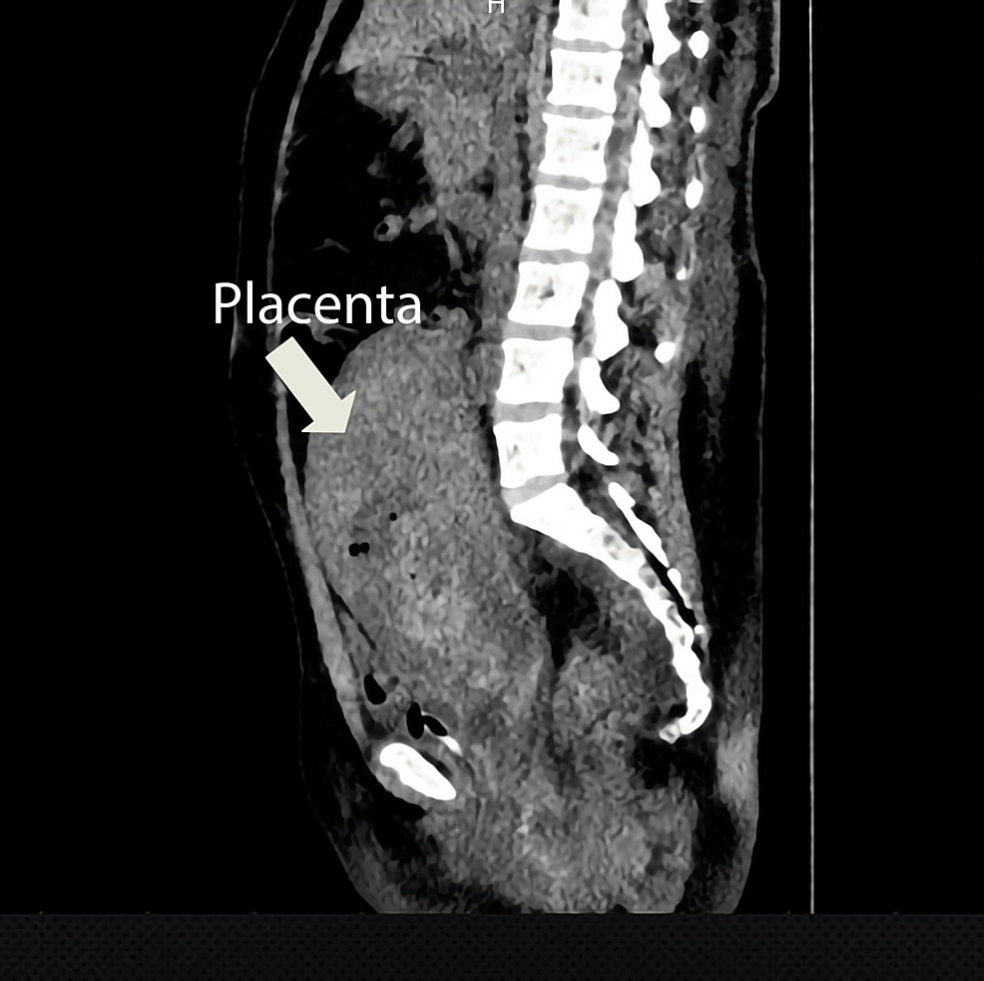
A sagittal computerized tomography (CT) view of the uterus, including the entrapped placenta
